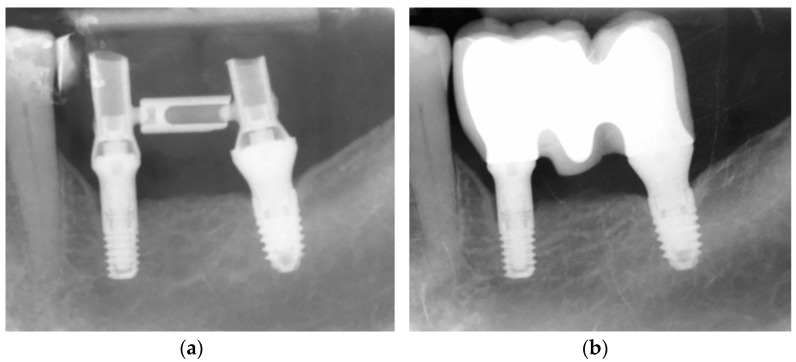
(a) Implant placement and immediate loading. #3.6 position implant: 3.0 mm diameter; 5.5 mm length; intermediate abutment height of 4.0 mm. #3.7 position implant: 3.75 mm diameter; 6.5 mm length; intermediate abutment height of 3.5 mm. (b) Final restoration one year after implant placement. (Periapical rx).
